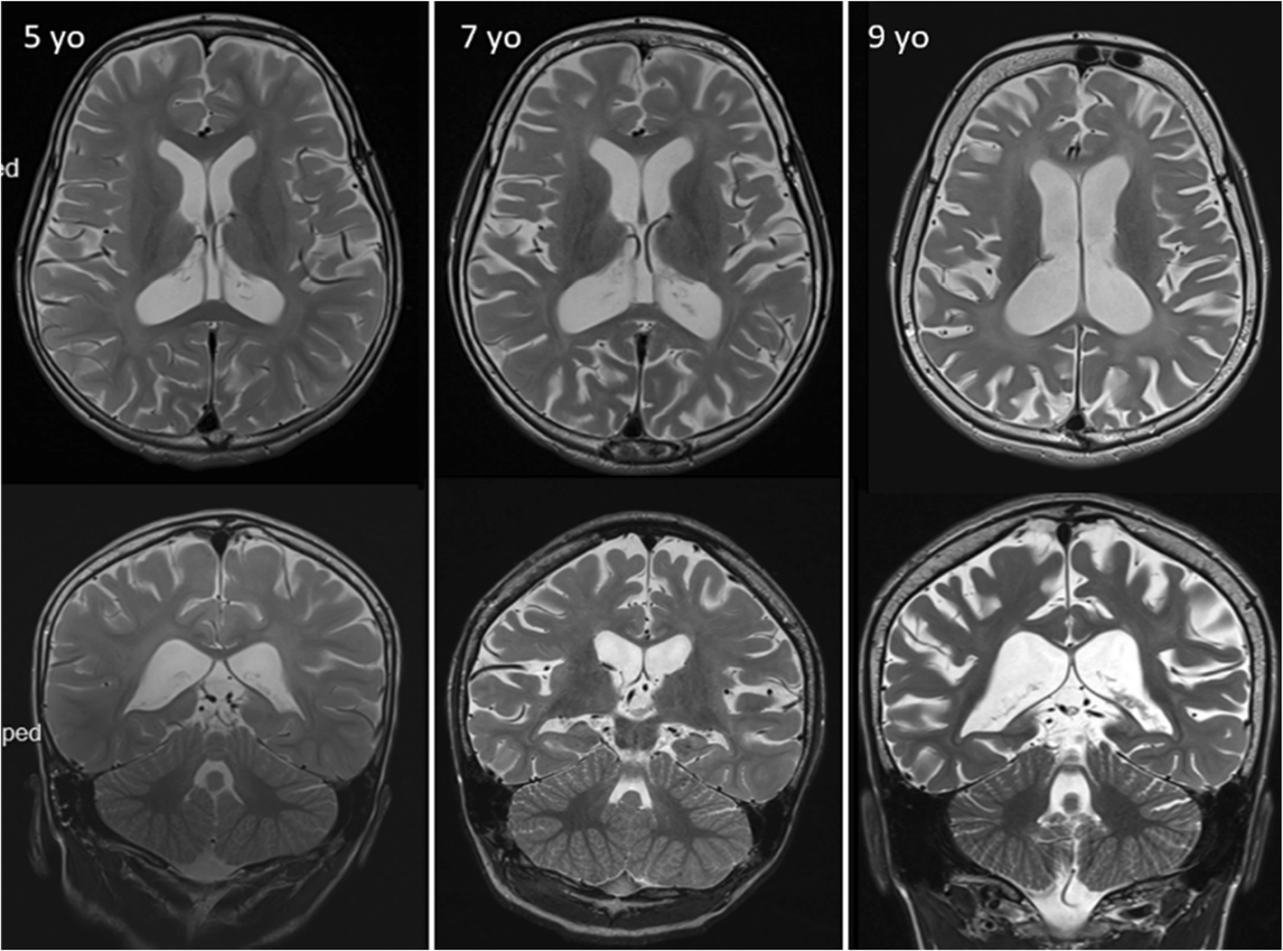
Brain MRI of the patient at different ages. Axial and coronal T2-weighted images. Supratentorial progressive cortical and subcortical atrophy with ex vacuo ventriculomegaly and diffuse deep white matter hyperintensities. Note that MRI is already abnormal at 5yo, before onset of regression. Cerebellar atrophy is only marginal. Basal ganglia, U-fibres, optic radiations, internal capsule and hippocampi were globally preserved
